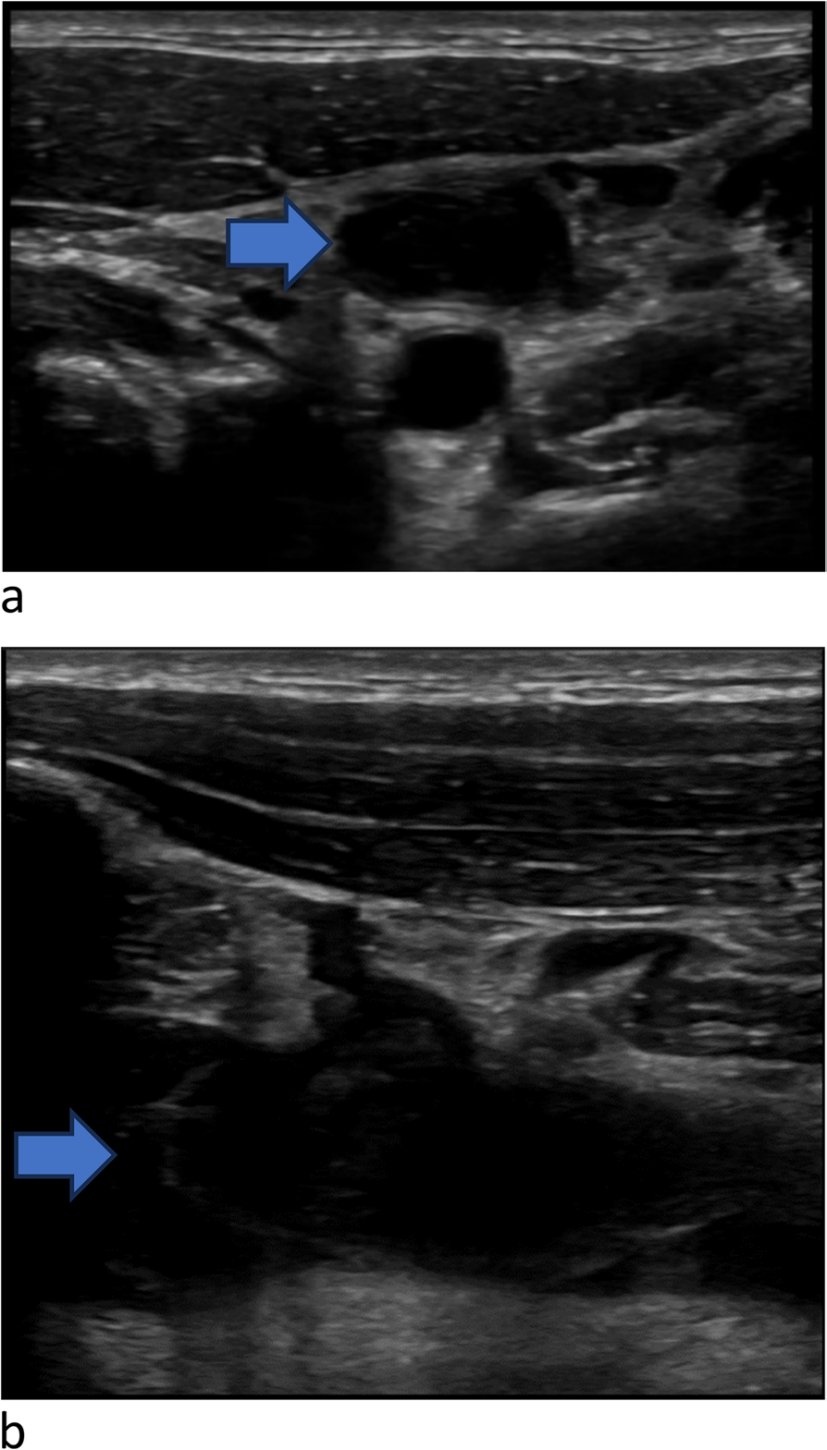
a Point-of-care ultrasound showing noncompressible left internal jugular vein (blue arrow) (b) Point of care ultrasound showing echogenic clot in the subclavian vein (blue arrow)
